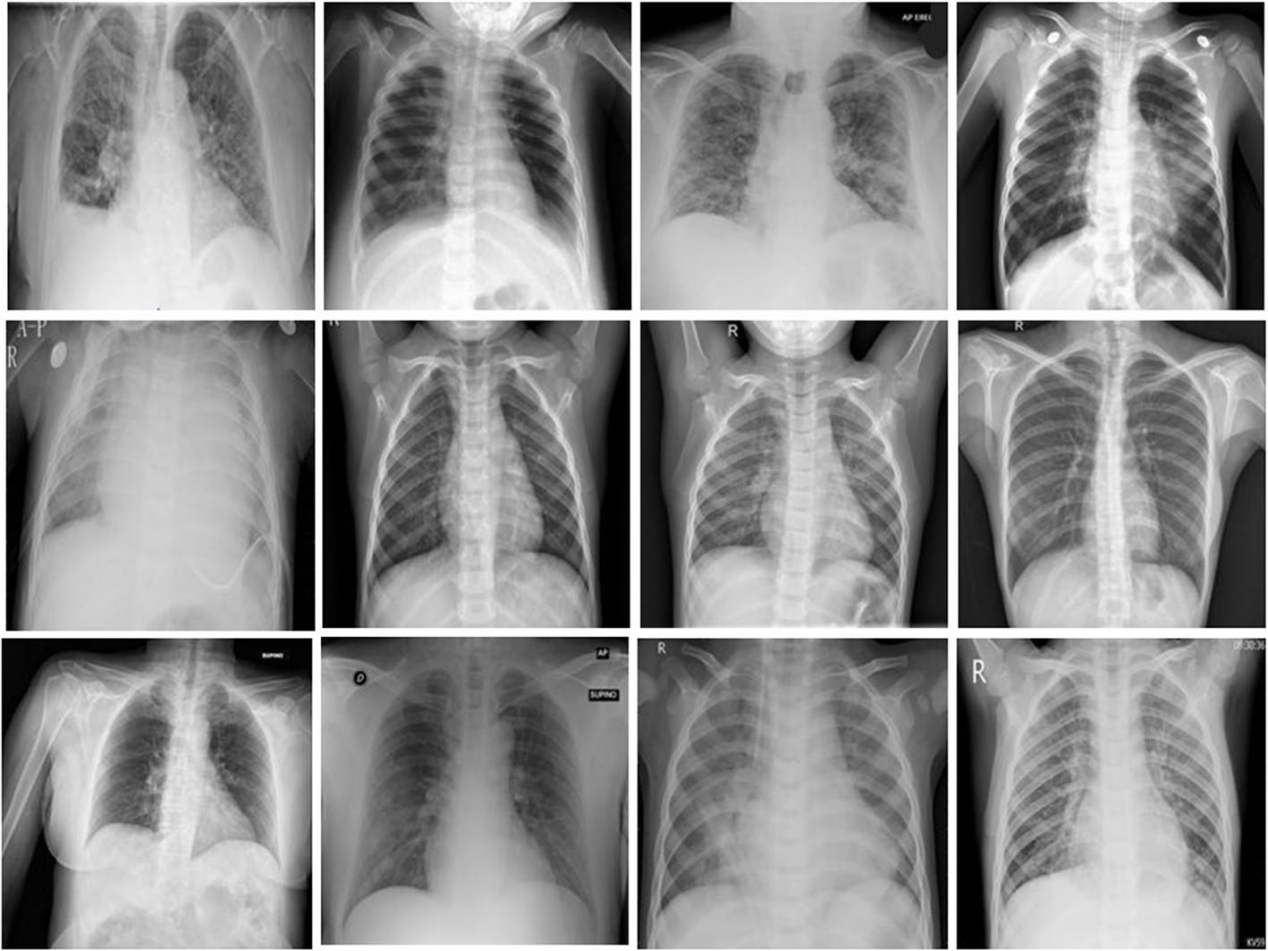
Random Samples of X-ray images of frontal chest cases from the DB^1^ to DB^6^ databases
